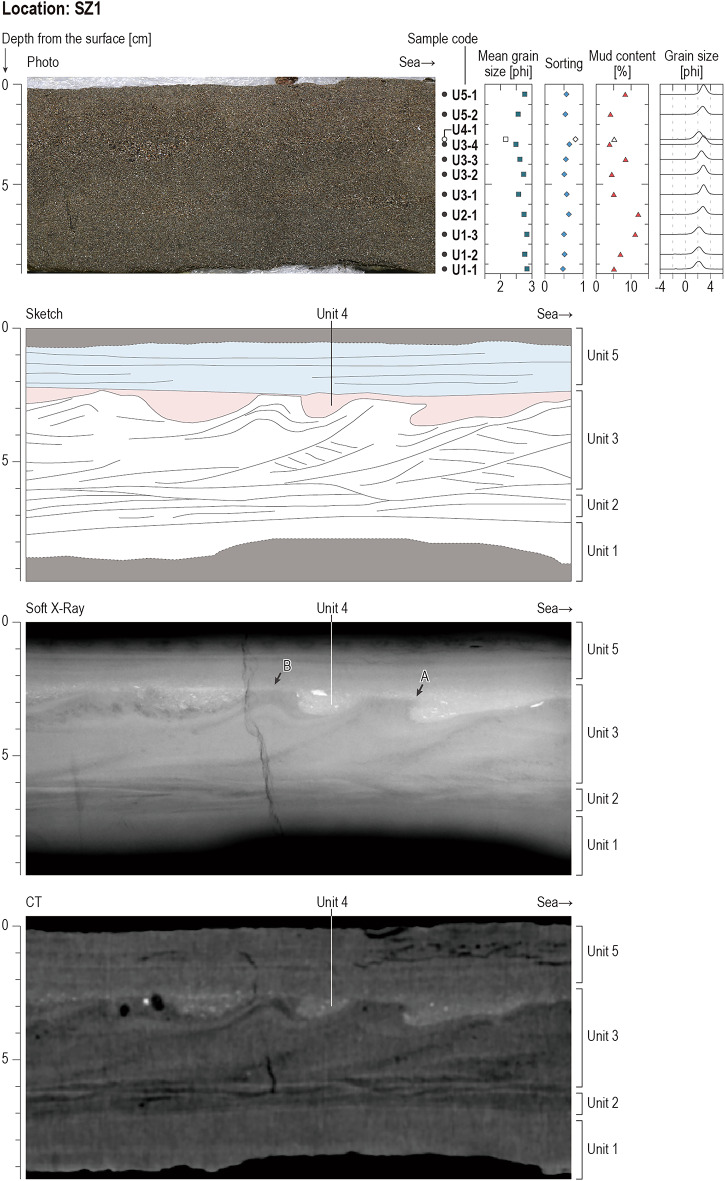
A photograph, a CT image, a soft X-ray image, a sketch, and graphs showing vertical changes in mean grain size, sorting, mud content, grain-size distribution at location SZ1. The mean grain size, sorting, mud content, and grain-size distribution of the sample from Unit 4 (U4-1) are represented by black symbols, to distinguish this sample from others from a similar depth. The specific sampling horizons from U1-1 to U5-2 are provided in the Supplementary Data S5.
